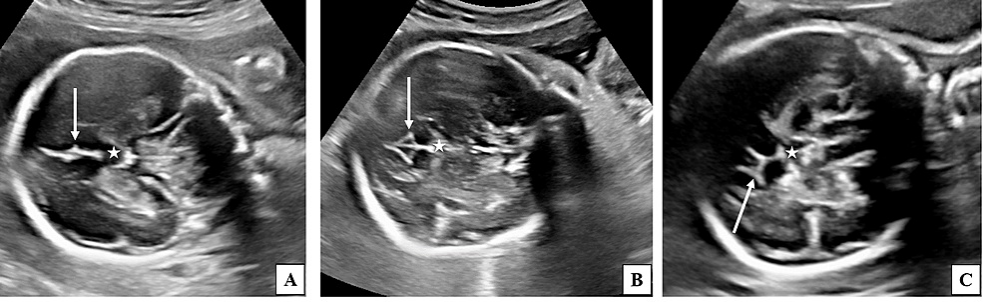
Two-dimensional ultrasound grey-scale images (coronal sections) where cavum septum pellucidum (star) was seen, demonstrating various patterns of CS at different gestational ages. (A) Fetus of 23 weeks 1 day gestational age demonstrating CS in the form of a dot pattern (long white arrow). (B) Fetus of 25 weeks 3 days gestational age demonstrating CS in the form of a V-shaped indentation (long white arrow). (C) Fetus of 28 weeks gestational age demonstrating CS in the form of a Y-shaped configuration (long white arrow). CS, cingulate sulcus
